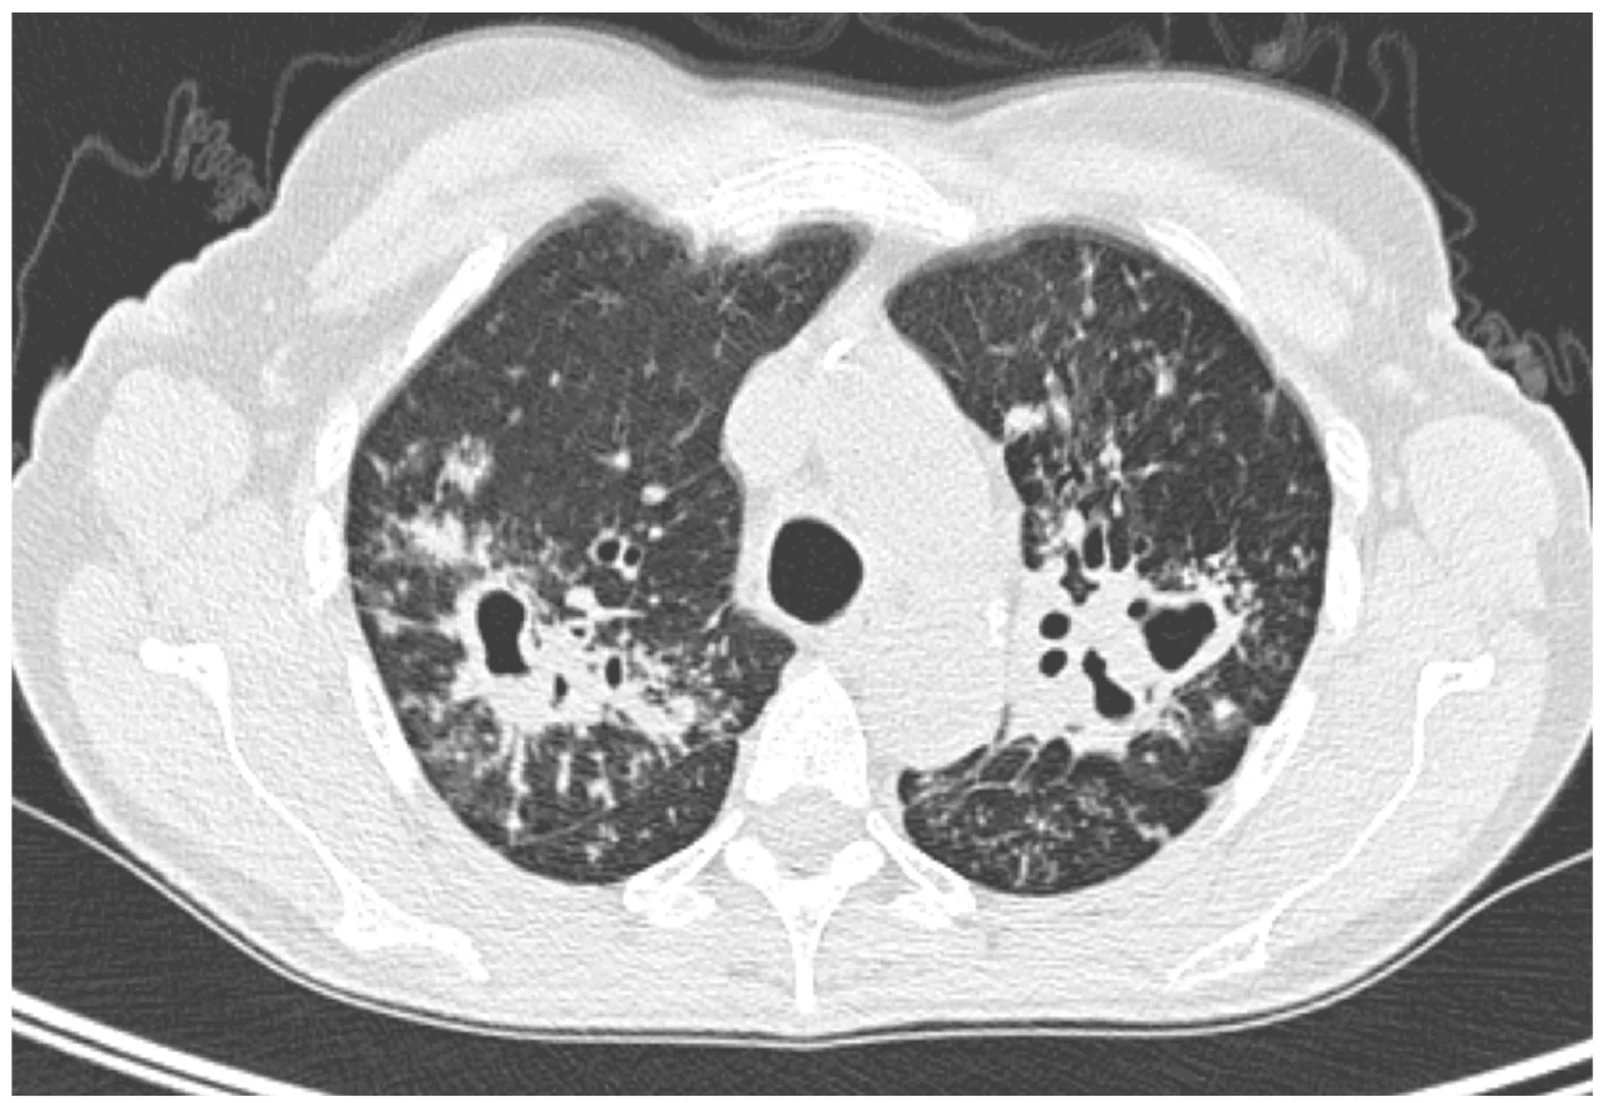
HRCT, axial plane. HRCT shows patchy heterogeneous consolidations involving posterior segments of the upper lobes, some of them cavitated, in a patient affected by reactivated TB.
